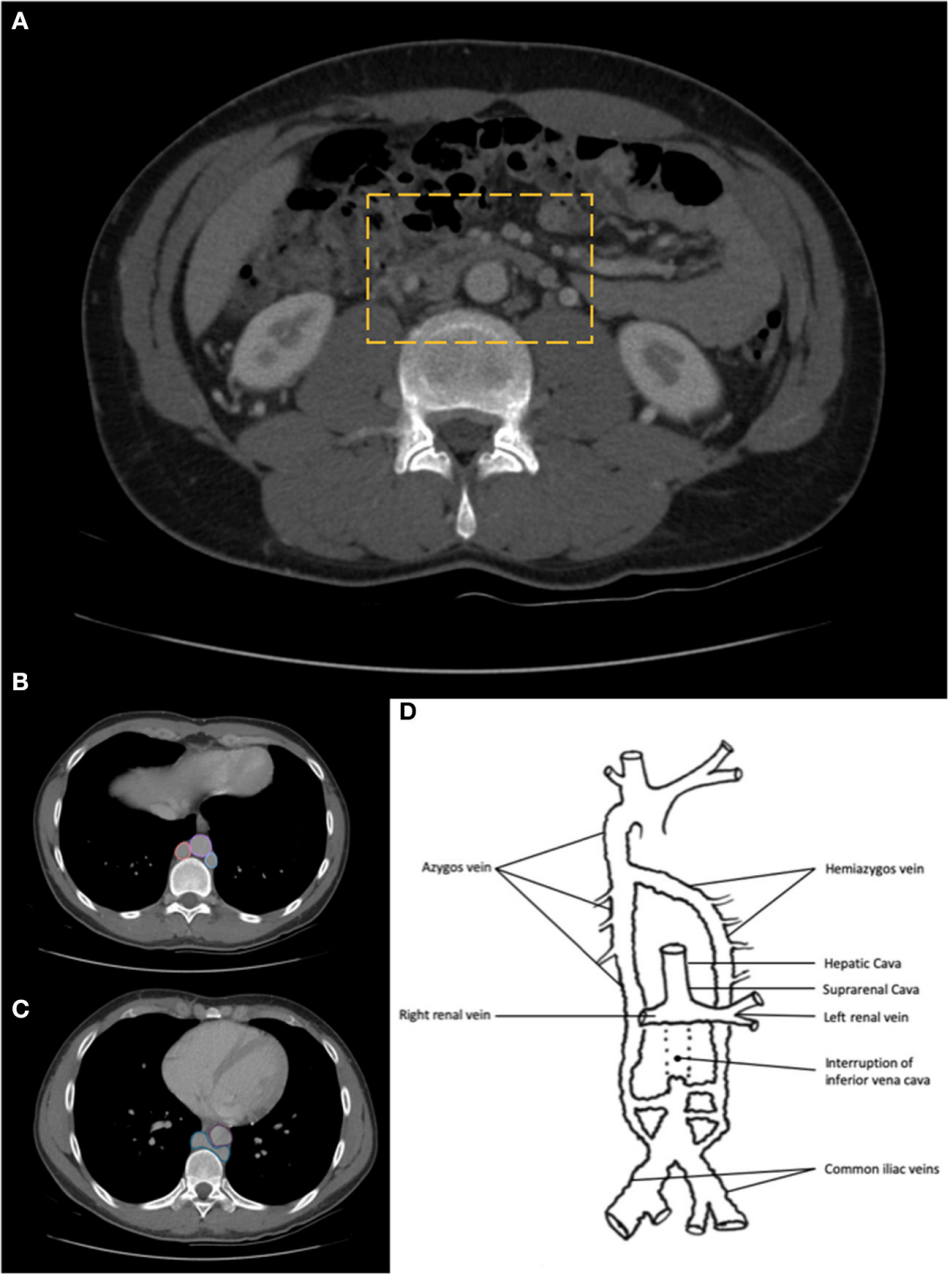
(A) CT-angiography reveals the complete absence of the infrarenal part of inferior vena cava (IVC). (B) Thorax CT-angiography highlights the role of the dilatation of azygos and hemiazygos systems. (Pink circle: Azygos vein; Violet circle: Aorta; Blue circle: Hemiazygos vein); (C) Thorax CT-angiography slice indicates the level of azygos and hemiazygos coalescence (blue shape). Aorta is highlighted by a purple circle; (D) Depicting the scheme of the anatomical patient's variant, highlighting the IVC agenesia and the development of the collateral venous drainage systems.
